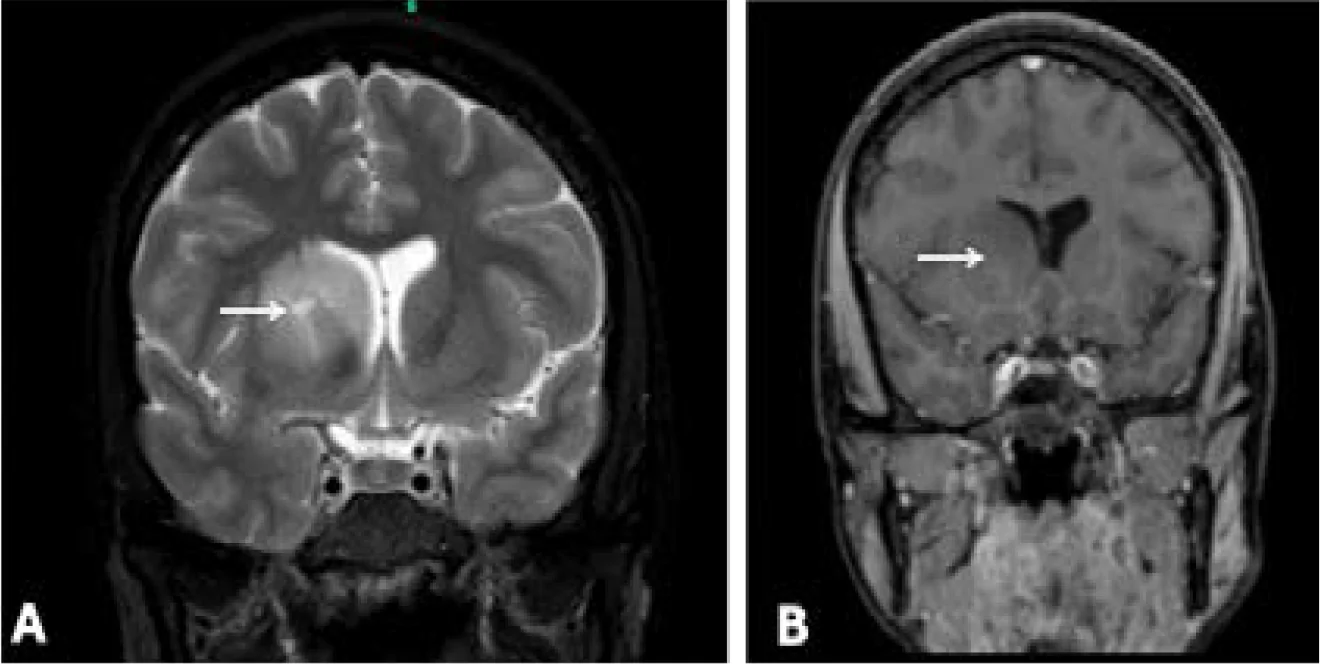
MRI of a 31-year-old woman with a one-week history of severe headache
